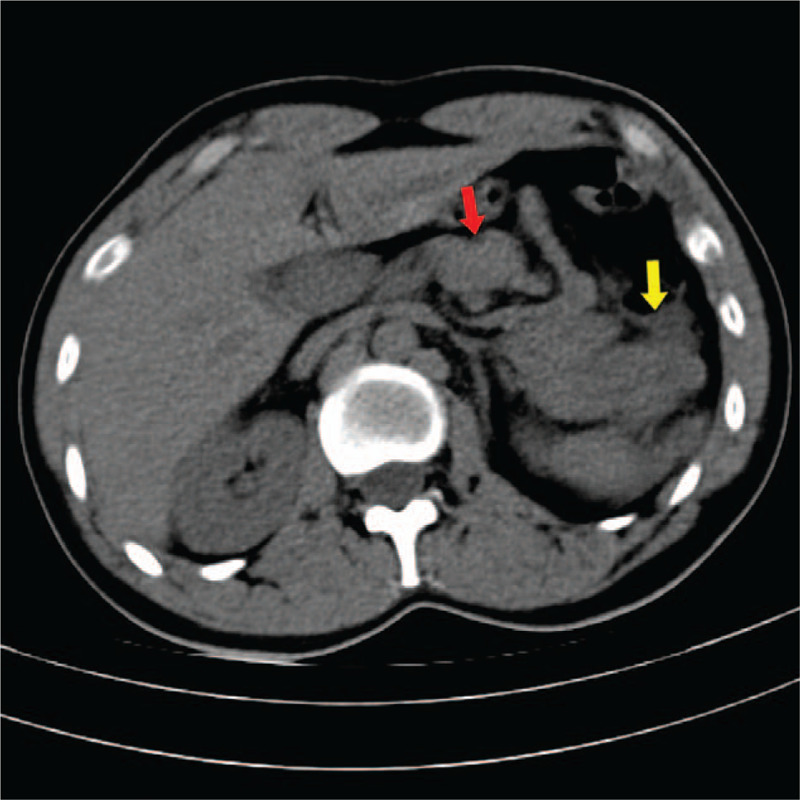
Sagittal CT image indicated the head of pancreas displaced into the left epigastrium, posterior to the left lobe of liver (red arrow), and fat stranding surrounding the swelled body and tail of the pancreas (yellow arrow). CT = computerized tomography.
